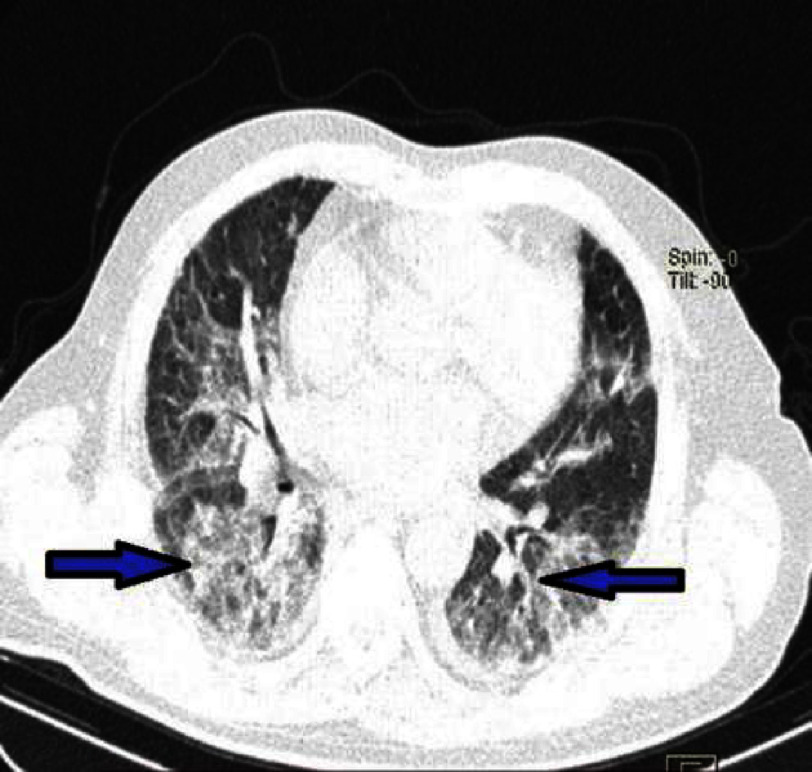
Chest CT scan showing predominantly centrilobular opacities at the bases on a lung parenchyma view (Patient #1).
